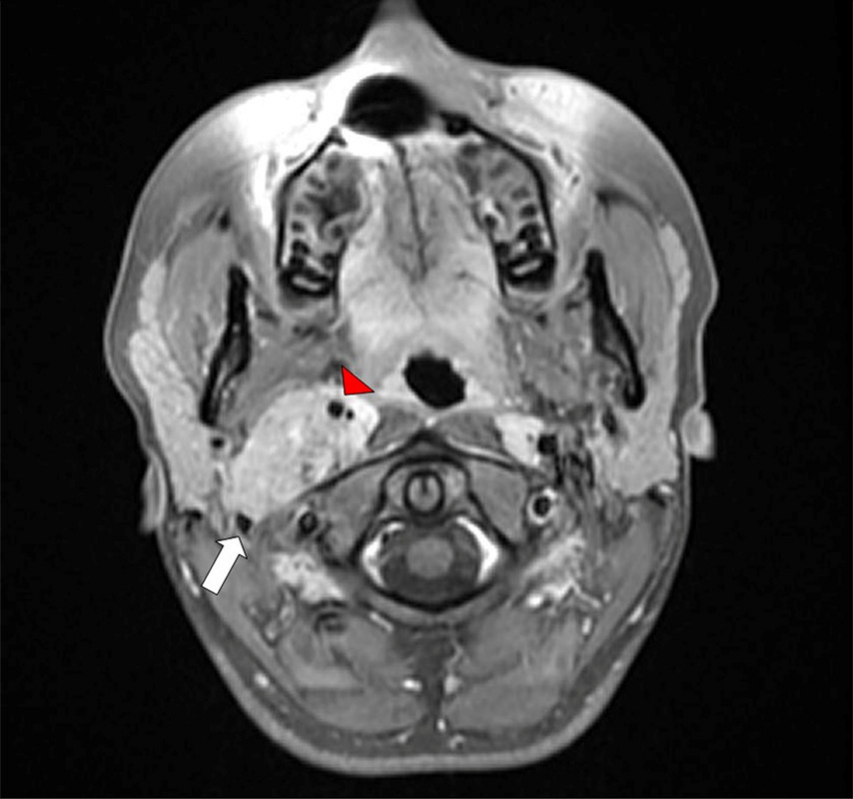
Axial *T*_1_ weighted MRI with contrast and fat suppression. Displacement of the internal carotid artery anteriorly (red arrow head) with the internal jugular vein pushed posteriorly (white arrow).
